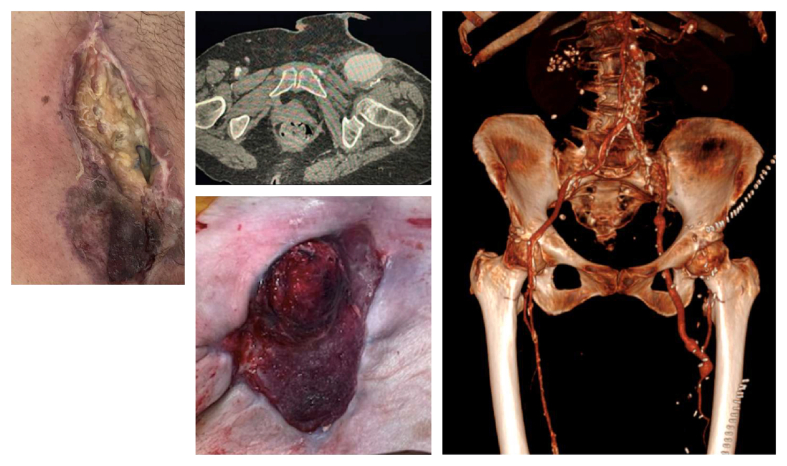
Pre-operative images showing typical clinical cases of femoral prosthetic skin erosion (left) and femoral pseudoaneurysm with cutaneous fistula (middle), treated with OFB. Post-operative 3D computed tomographic angiography reconstruction after a left OFB for an infected femoral pseudoaneurysm (right).
